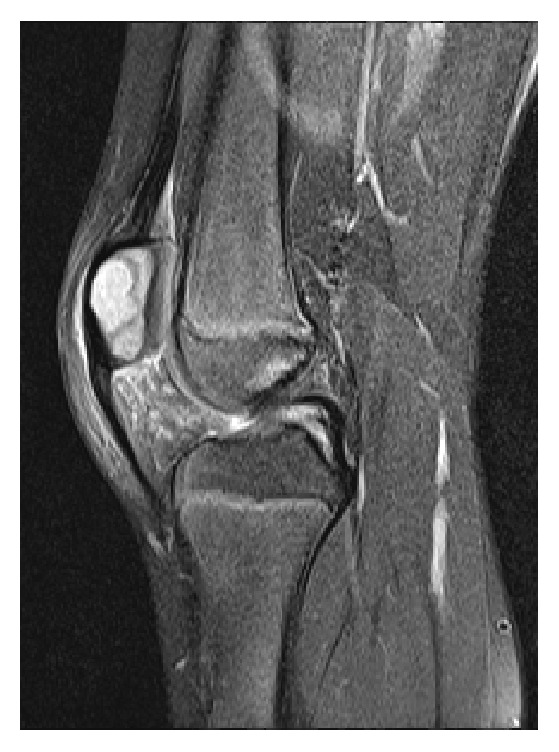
MRI showing a sharply limited enhancement of the patella. The radiologist suspected a chondroblastoma.
